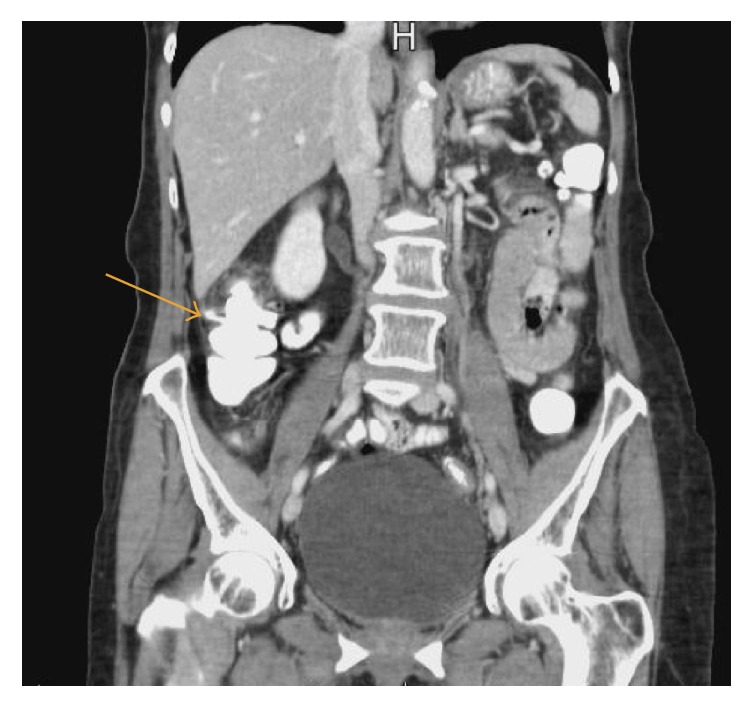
CT scan (coronal view) showing an extension of contrast from the right posterior colon, cranial to the appendix.
